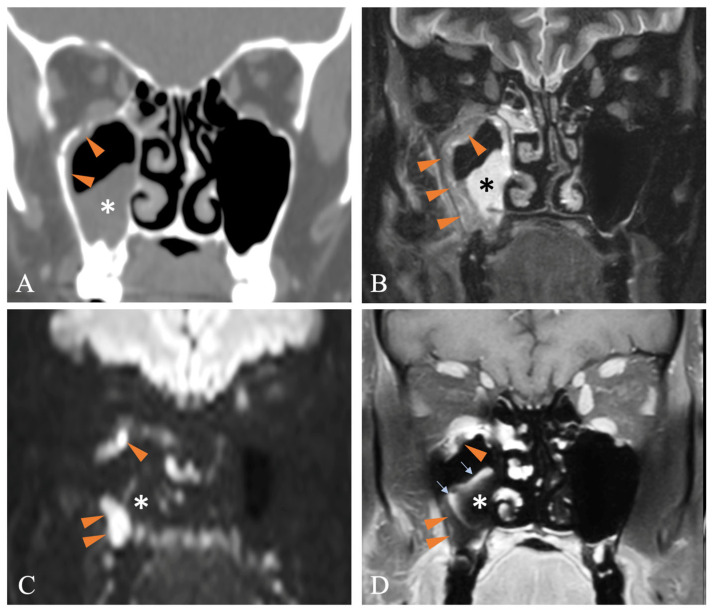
54-year-old male confirmed with right AIFR (*Mucor*). Coronal CT (A) and corresponding T2W fat-saturated (B), diffusion-weighted (C), and contrast-enhanced T1W fat-saturated (D) MR images. On CT, there is non-specific opacification of the right maxillary sinus (asterisk) and minimal mucosal thickening along the lateral sinus wall and roof (arrowheads). On MRI, the mucosa covering the roof and lateral sinus wall appears slightly hypointense on the T2W image (arrowheads in (B)), shows restricted diffusivity (arrowheads in (C)), and the vast majority does not enhance (arrowheads in (D)). The non-specific opacification seen in (A) corresponds to an inflammatory polyp (large asterisks on (A–D)) characterized by a very high signal on the T2W image, no restriction of diffusivity, and only thin mucosal superficial enhancement (thin arrows in (D)).
